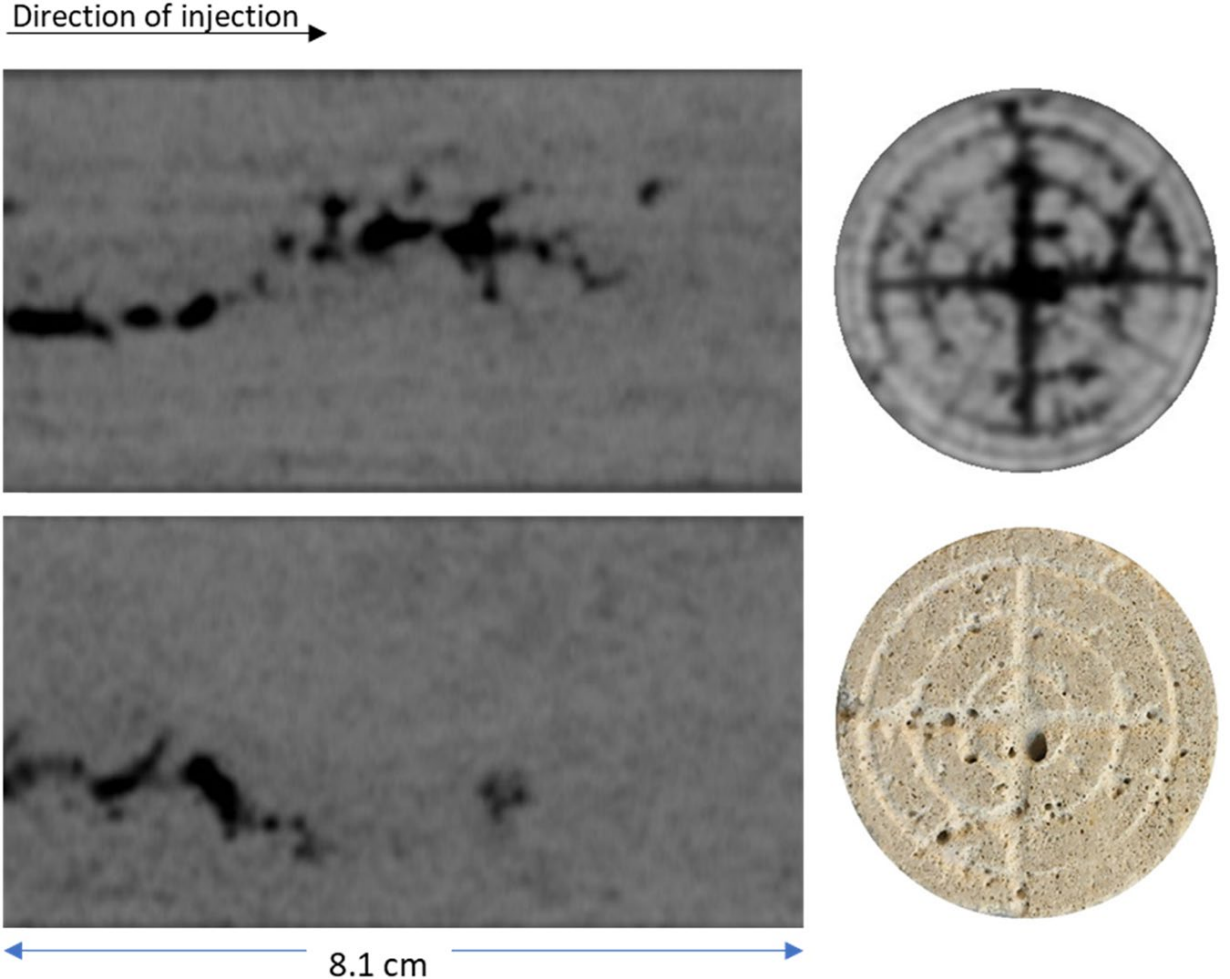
X-ray CT images of the Core A after carbonated brine injection. Two orthogonal longitudinal views from the inlet (left) to the outlet (right) and a transversal view at the near inlet face of the core.
